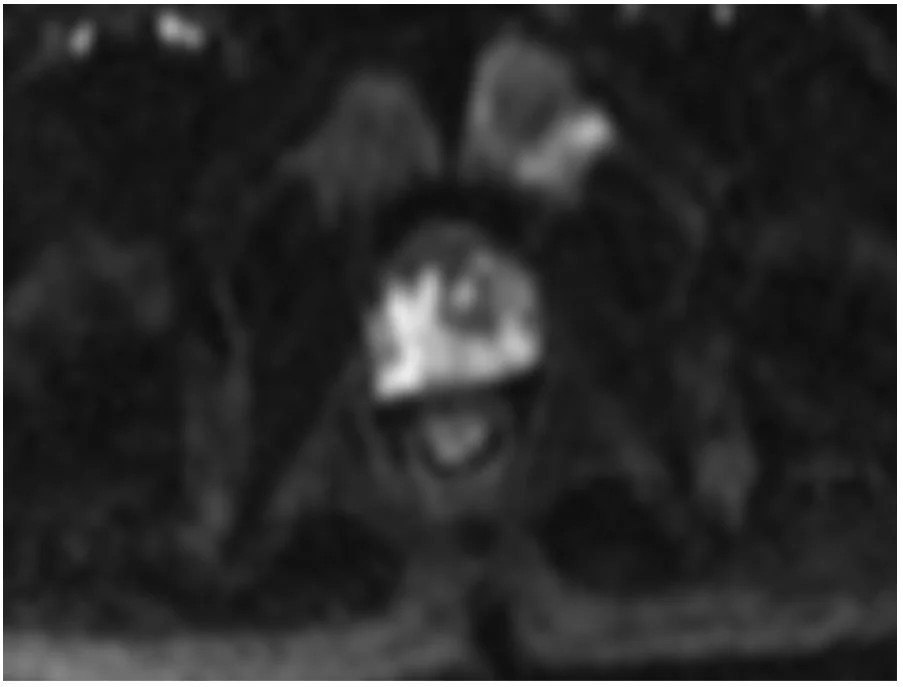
Prostate mpMRI with DWI was performed in a 67-year-old man with bone metastasis. MRI, magnetic resonance imaging; DWI, diffusion-weighted imaging.
